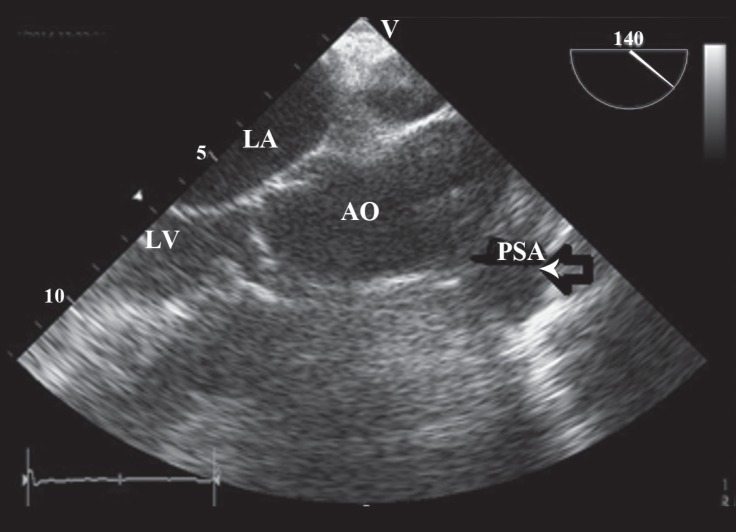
Preprocedural transesophageal echocardiography, parasternal long-axis view, shows the pseudoaneurysm of the aorta (arrow).
